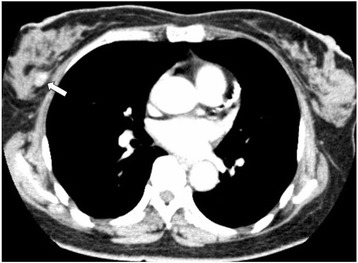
Computed tomography of the chest showing the right breast nodule with irregular margins
